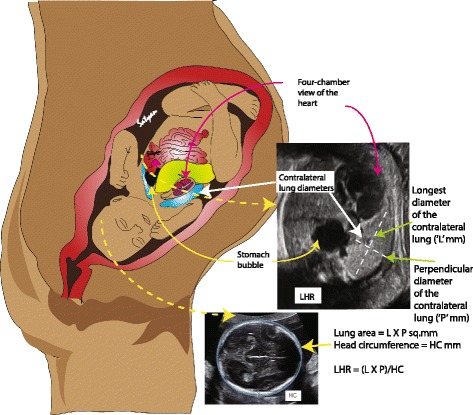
Lung to head ratio (LHR) measurement – Obstetric ultrasound technique is used to measure the lung to head ratio known as LHR to assess the severity of CDH. The head circumference is measured as shown. The contralateral lung area is calculated as a product of the longest and perpendicular diameter of the contralateral lung. The ratio of this area to the head circumference gives the LHR. A LHR of <0.6 has been associated with poor outcome while a ratio of >1.35 has been associated with survival. Alternatively, an observed to expected LHR measurement is used in order to overcome bias secondary to gestational age. *(Copyright Satyan Lakshminrusimha)*
